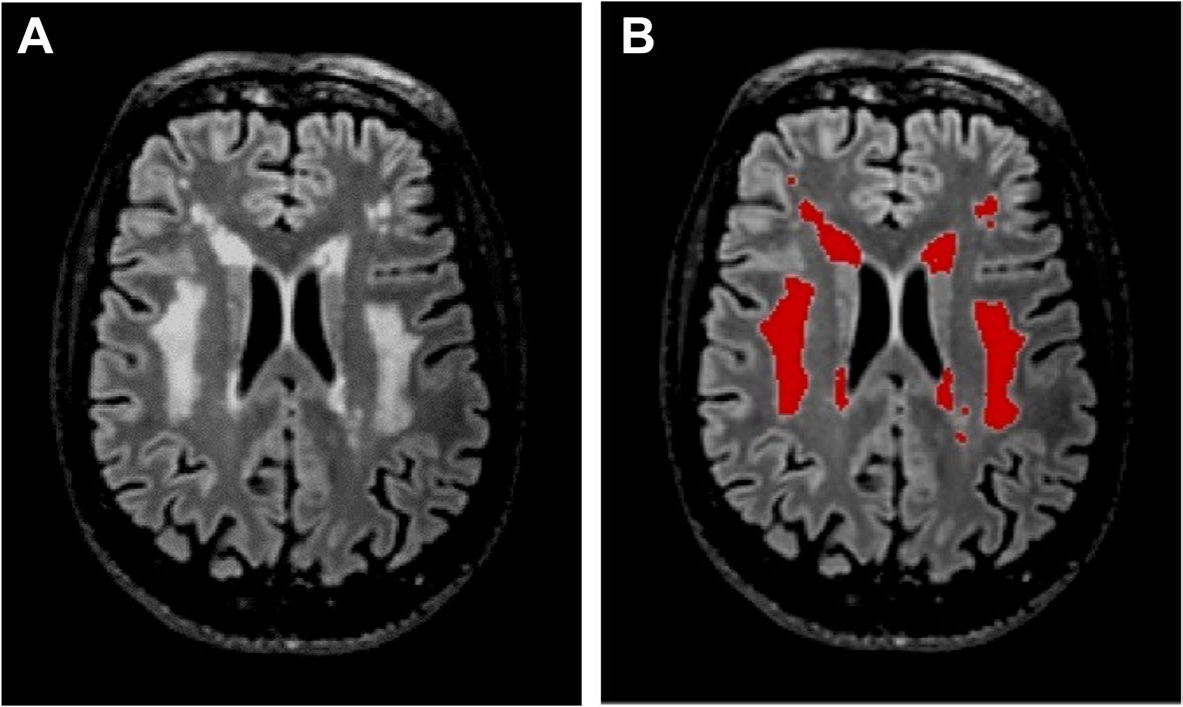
Representative MRI T2-weighted images from a 68-year-old male with SCD showing A substantial WMH damage (Fazekas scale score = 3) and B isolation and masking of the WMH burden (in red) using the PGS software. Abbreviations: MRI: magnetic resonance imaging; WMH: white matter hyperintensitiy; SCD: subjective cognitive decline
